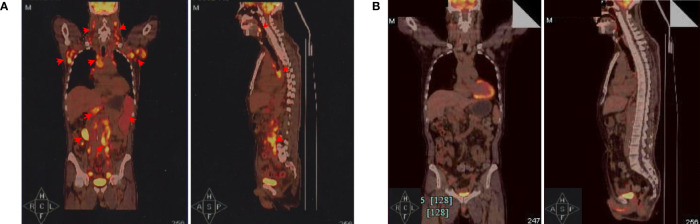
Positron emission tomography and computed tomography (PET-CT) evaluations. (A) PET-CT before CAR-T cell infusion. The coronal image (left) and sagittal image are shown (right). Red arrows indicate lymphoma lesions. (B) PET-CT examination showed significantly diminished lymphoma lesions and proved complete metabolic remission (CMR) 3 months after CAR-T cell therapy.
